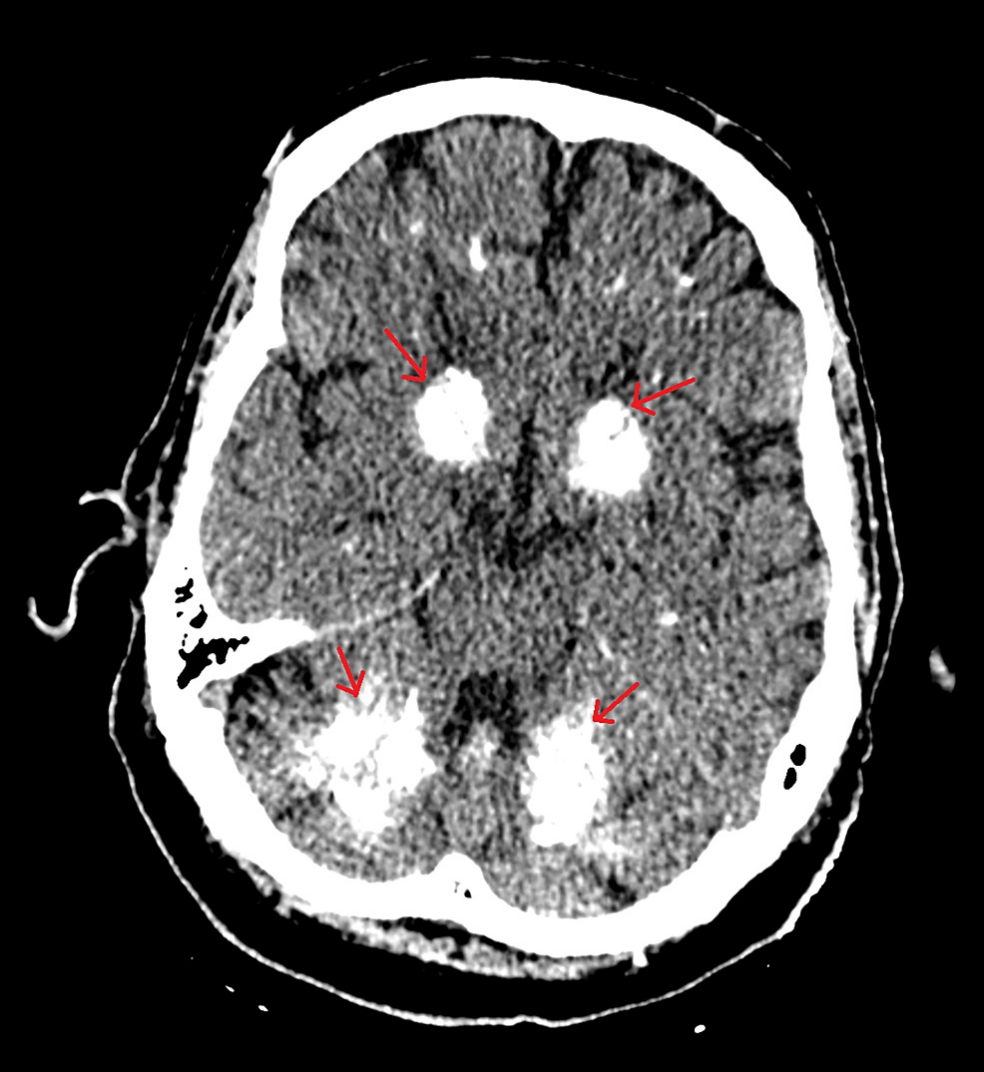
CT of the brain showing bilateral calcifications
